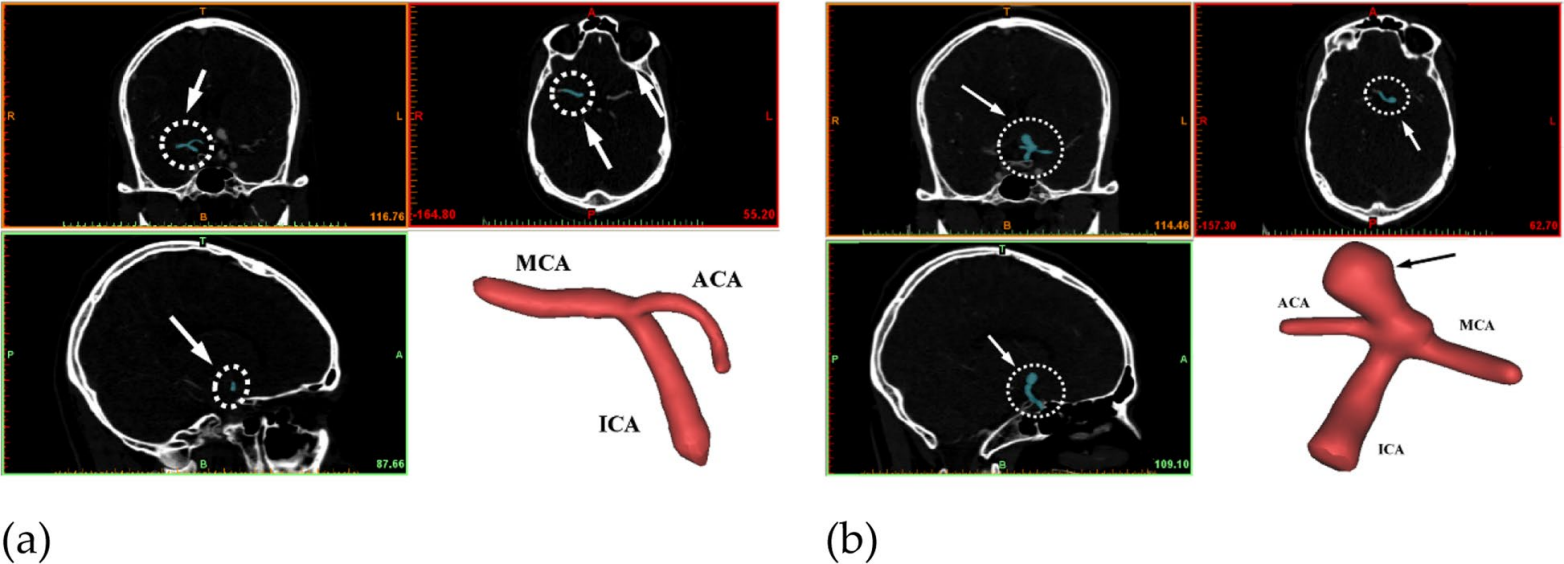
Different views of CT scan of ICA bifurcation. a: Normal; b: Giant aneurysm
